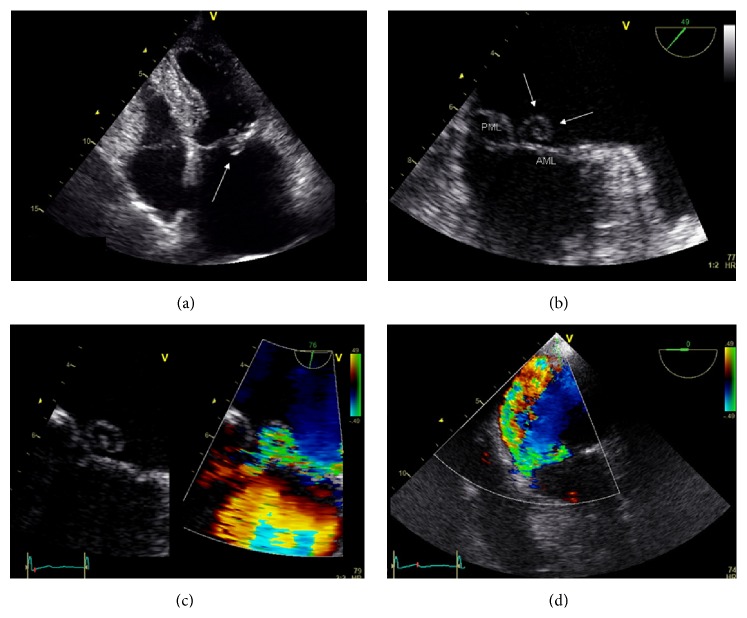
(a) Transthoracic echocardiography showing a rounded-shaped mass attached to a prolapsing posterior mitral leaflet. (b) Transesophageal echocardiography revealing a spiral-like appearance of the mass. (c) Color Doppler image showing high turbulence within the vegetation. (d) Color-Doppler image showing severe mitral regurgitation.
